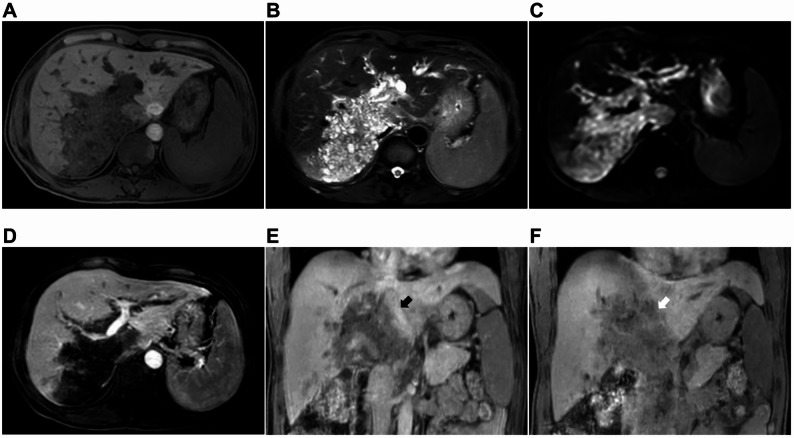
MRI findings. (A, B,C) The MRI plain scan revelaed a substantial lesion in the right posterior lobe of the liver and porta hepatis, appearing hypointense on T1WI and hyperintense on T2WI and DWI. The lesion showed periportal infiltration, encasing the right and left portal veins, and invading of the caudate lobe. (D) The enhanced MRI demonstrated no enhancement of the lesion during the arterial phase. (E, F) Mild local enhancement (black arrow) was observed at 3 min after contrast agent injection; however, the lesion exhibited hypointensity (write arrow) at 15 min post-injection
